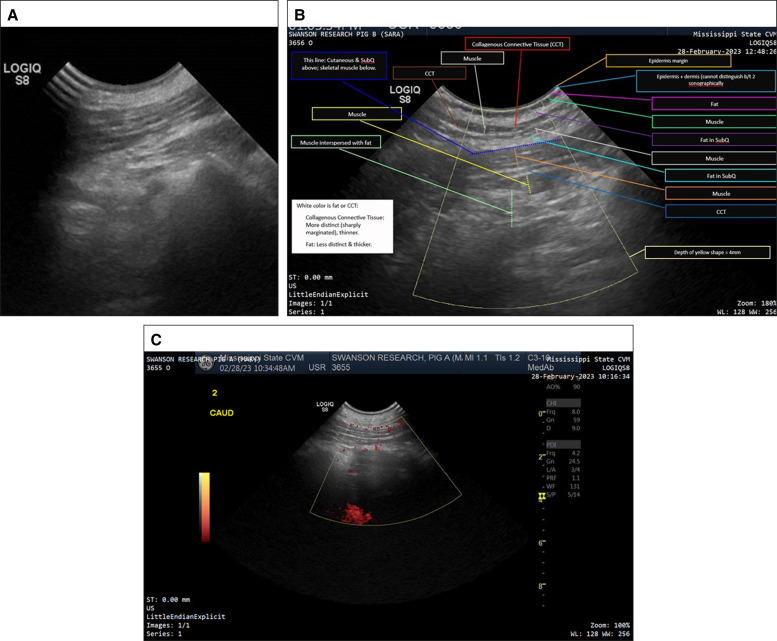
(A) Ninety days postoperative, diagnostic ultrasound image reveals no nodules, cysts, fluid collections, or tissue separations (as well as the remainder of the 16 dissected sites). (B) Labeled ultrasound layers display the normal, multilevel interpolation of numerous muscular bands throughout the subcutaneous fat. Note the labeling of the areas. (C) Ninety days postoperative in vivo Doppler ultrasound reveals slightly enhanced vascularity as per ultrasonographer and algorithm quantification.
